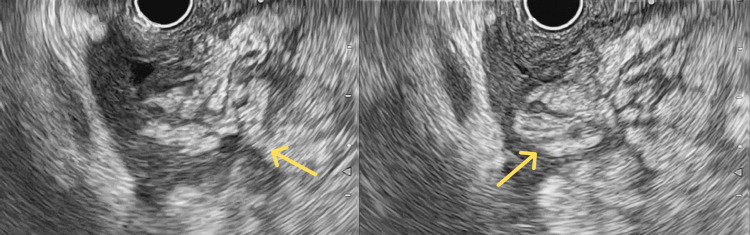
Endoscopic ultrasound Endoscopic ultrasound showing a hyperechoic septated mass affecting a major portion of the left hepatic lobe
